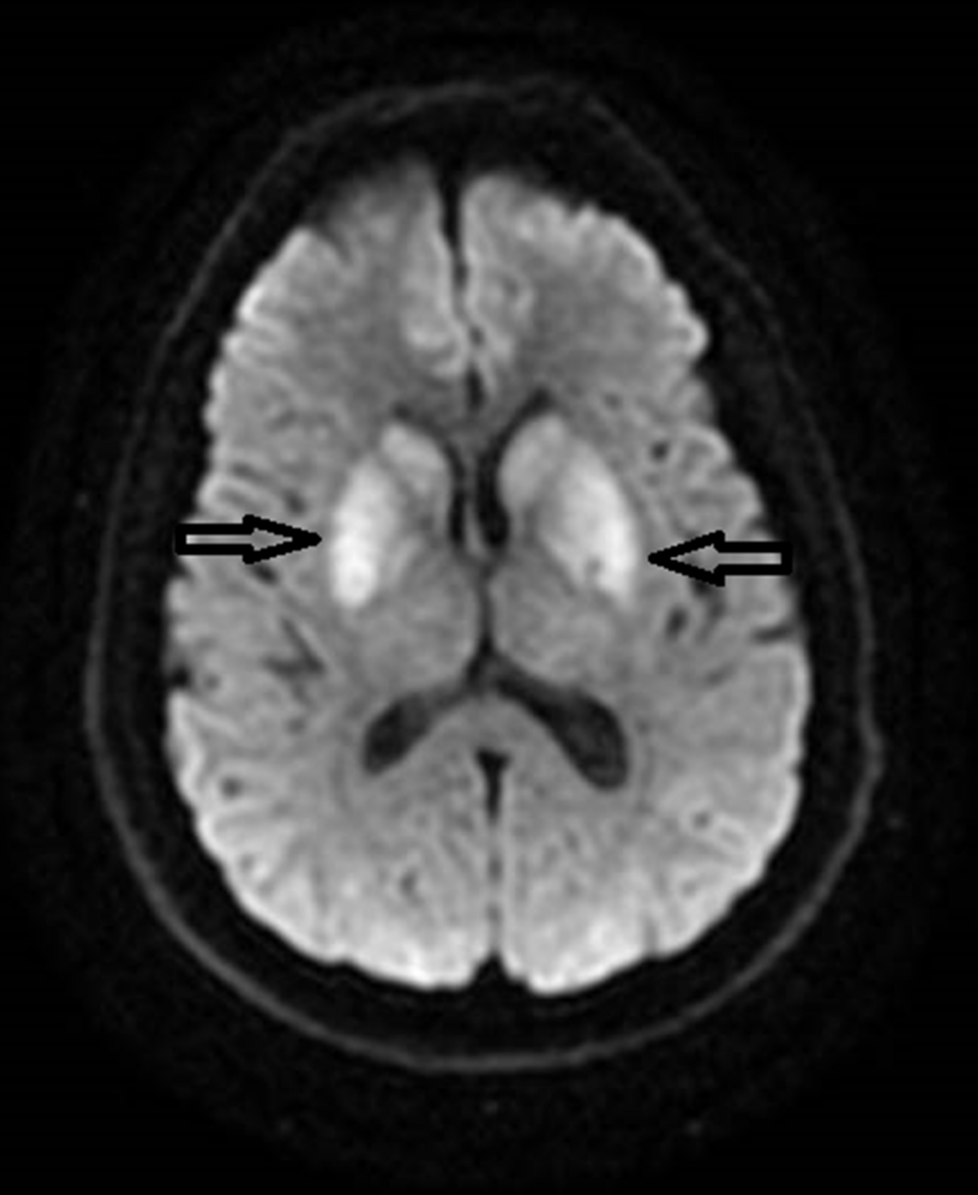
MRI brain axial DWI showing restricted diffusion in bilateral basal ganglia. MRI: Magnetic resonance imaging; DWI: Diffusion weighted imaging.
